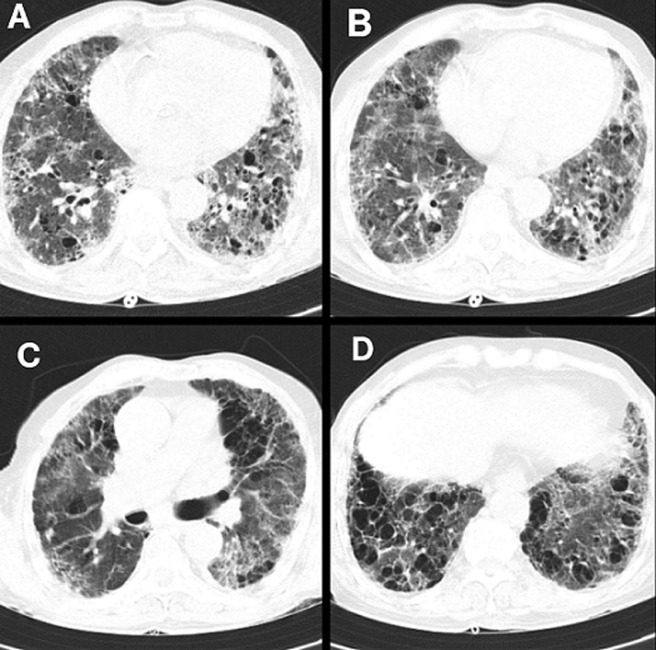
HRCT scan of the thorax, (A and C) tractional bronchiectasis and multiple fibrotic changes in bilateral lung fields showing apicobasal gradient, with (D) honeycombing pattern and (B) patchy areas of consolidation and thickening of the septa in bilateral lung fields
